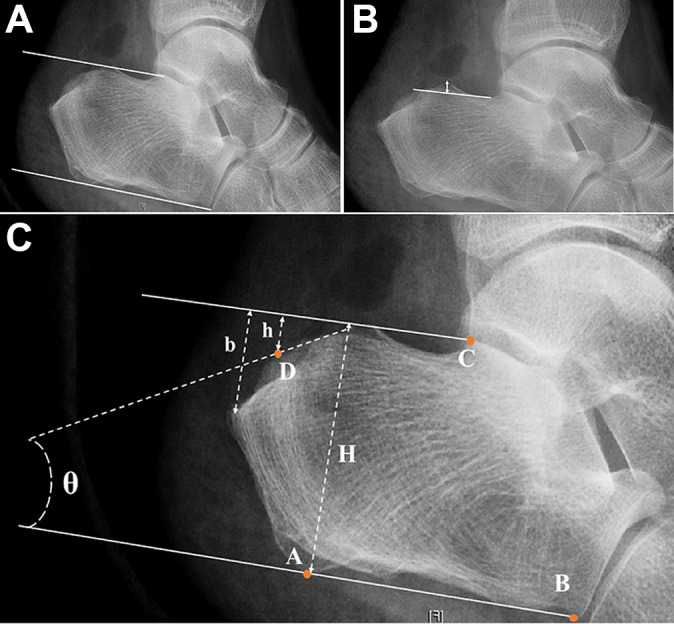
Radiographic parameters on postoperative radiographs. (A) Pitch line. (B) Haglund deformity height (double-headed arrow). (C) The calcaneal height ratio (CHR), calcaneal resection ratio (CRR), and calcaneal resection angle were measured as follows. The following landmarks were identified: the most inferior point of the posterior tuberosity, *A*; the inferior edge of the anterior calcaneus at the calcaneocuboid joint, *B*; the inferior parallel pitch line, line *AB*; the most posterior extension of the superior pitch line in panel (A), *C*; the exit point of the osteotomy from the posterior calcaneal tuberosity, *D*; the distance from the superior pitch line to the point of Achilles insertion, *b*; and the distance from the superior pitch line to point *D*, *h*. These landmarks were used to calculate the CHR (ratio of *h* and *H*), CRR (ratio of *h* and *b*), and calcaneal resection angle (θ; between the inferior pitch line and a line of best fit along the calcaneal resection).
